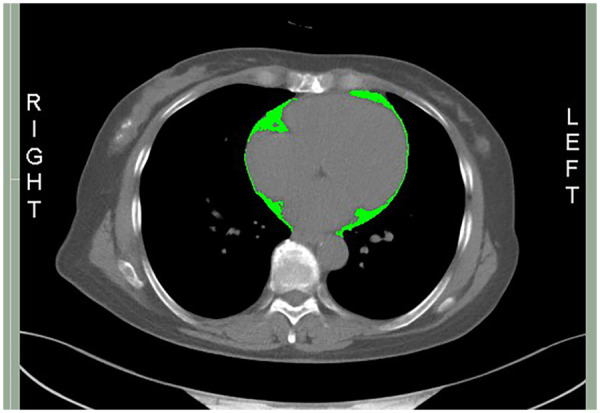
Epicardial adipose tissue. The epicardial fat area (EFA, highlighted in green) was quantified using chest computed tomography (CT) images.
